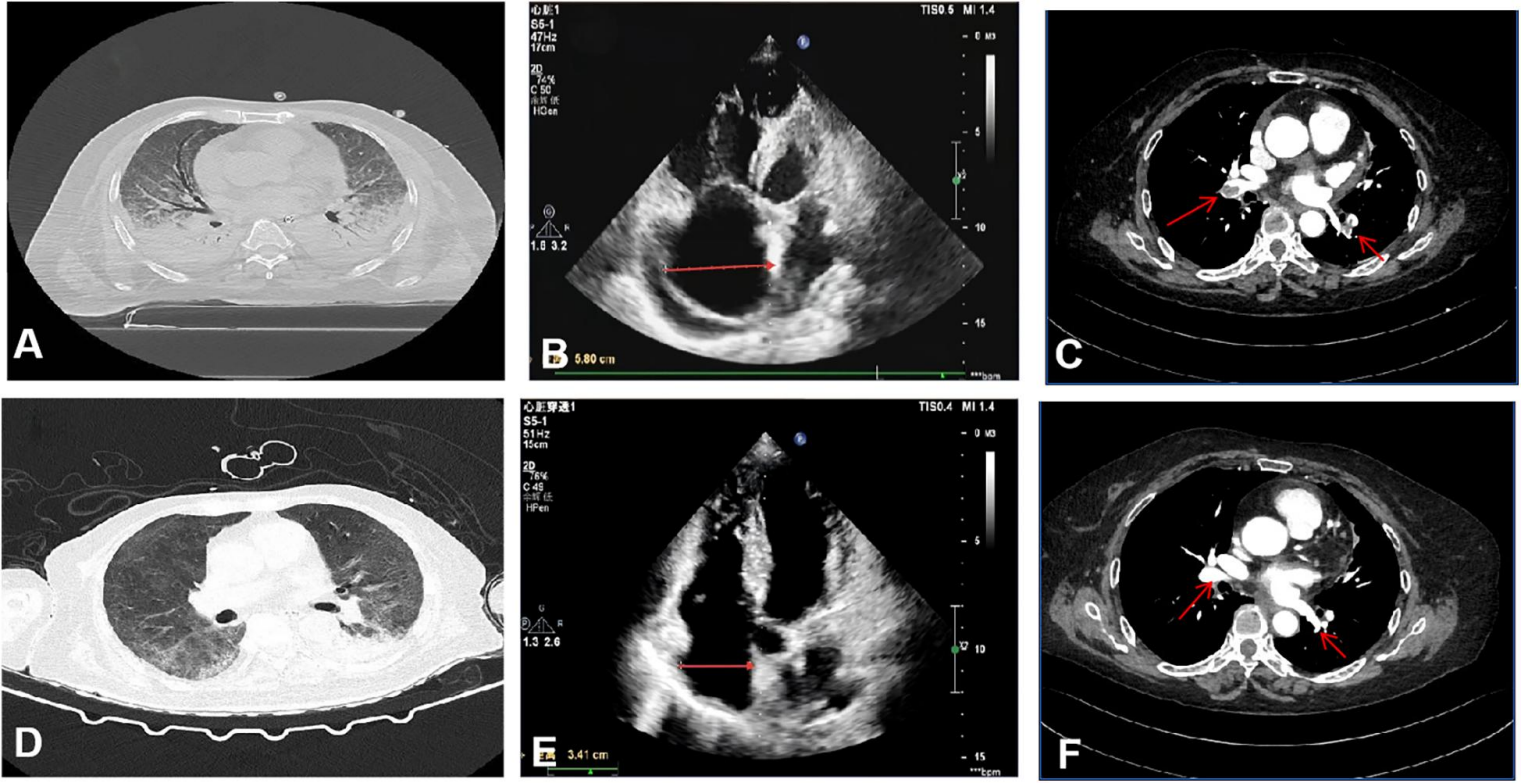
Imaging and echocardiographic findings before and after treatment. (A–C) Pre-treatment: (A) Chest CT shows bilateral pulmonary consolidation and interstitial infiltrates. (B) Transthoracic echocardiography reveals right atrial enlargement (transverse diameter 5.8 cm). (C) CT pulmonary angiography demonstrates extensive bilateral pulmonary embolism. (D–F) Post-treatment: (D) Chest CT indicates resolution of pulmonary consolidation and marked improvement of interstitial opacities. (E) Echocardiography shows significant reduction in right atrial size (transverse diameter 3.41 cm). (F) CT pulmonary angiography confirms substantial reduction in thrombus burden.
